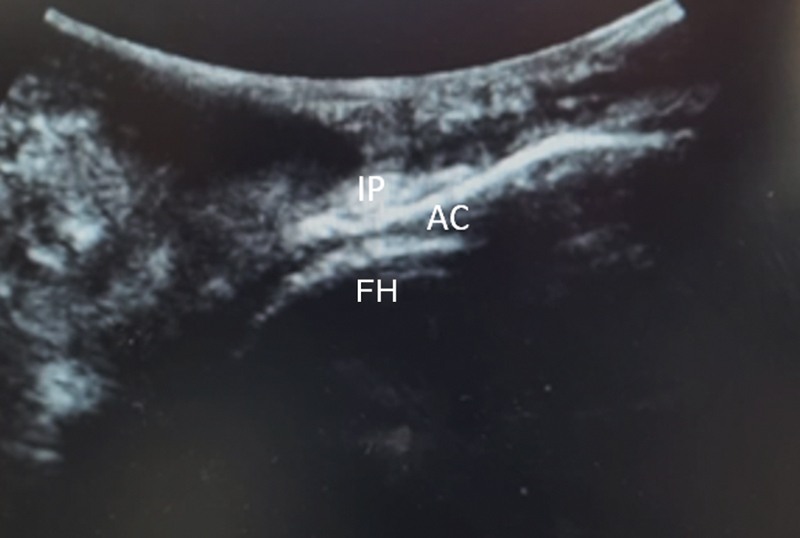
Ultrasound view of the “stacking sign” with the transducer transverse to the frontal plane showing the femoral head, the superior acetabular rim, and the iliopsoas tendon.
